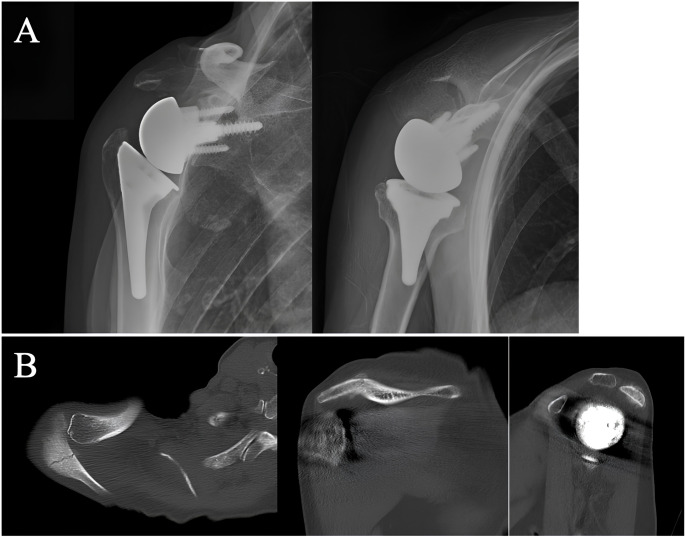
69-year-old female with new shoulder pain three months after primary rTSA, with unrevealing AP and Velpeau x-rays (A), found on CT to have a nondisplaced Levy zone IIA acromial stress fracture (B). Pain resolved with eight weeks of nonoperative management; range of motion deficits persisted but were well-tolerated
